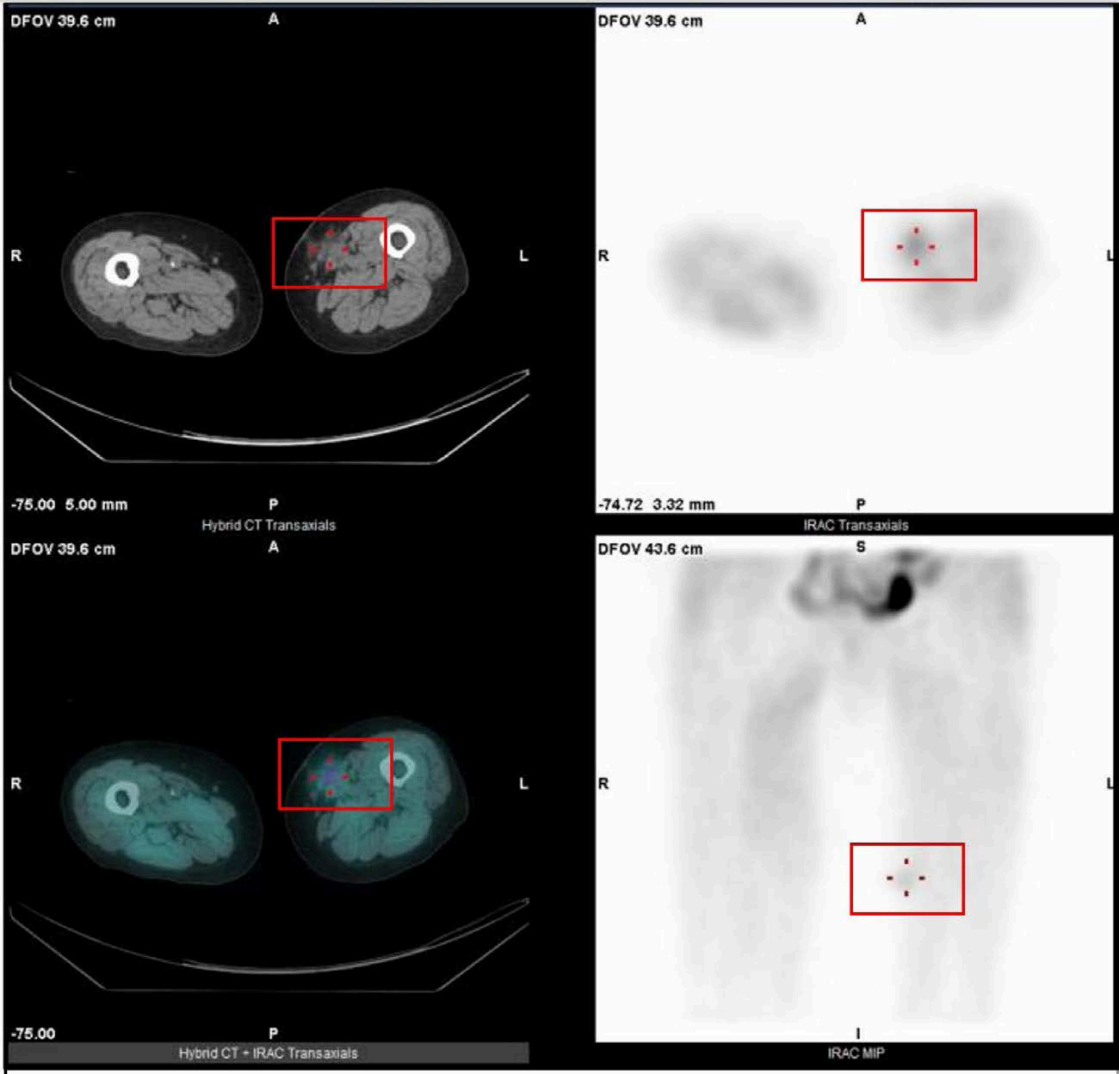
SPECT showed accurate position abnormal uptake at subcutaneous layer at lower medial aspect of left thigh. (square frame).
